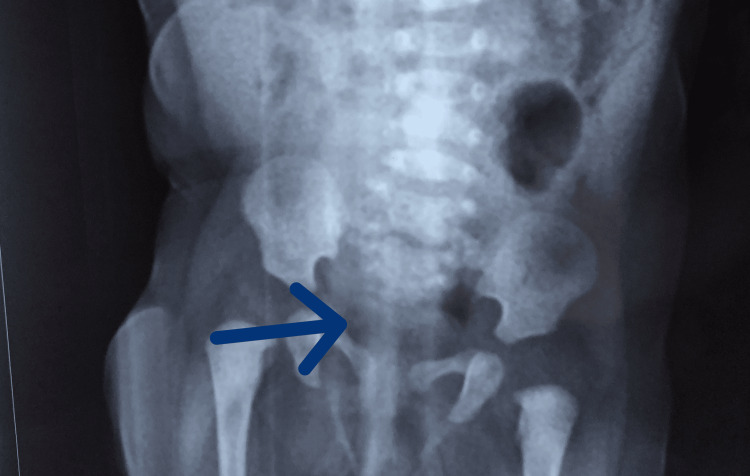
X-ray showing partial agenesis of the fourth and fifth sacral vertebrae. The blue arrow points to the sacrum.
